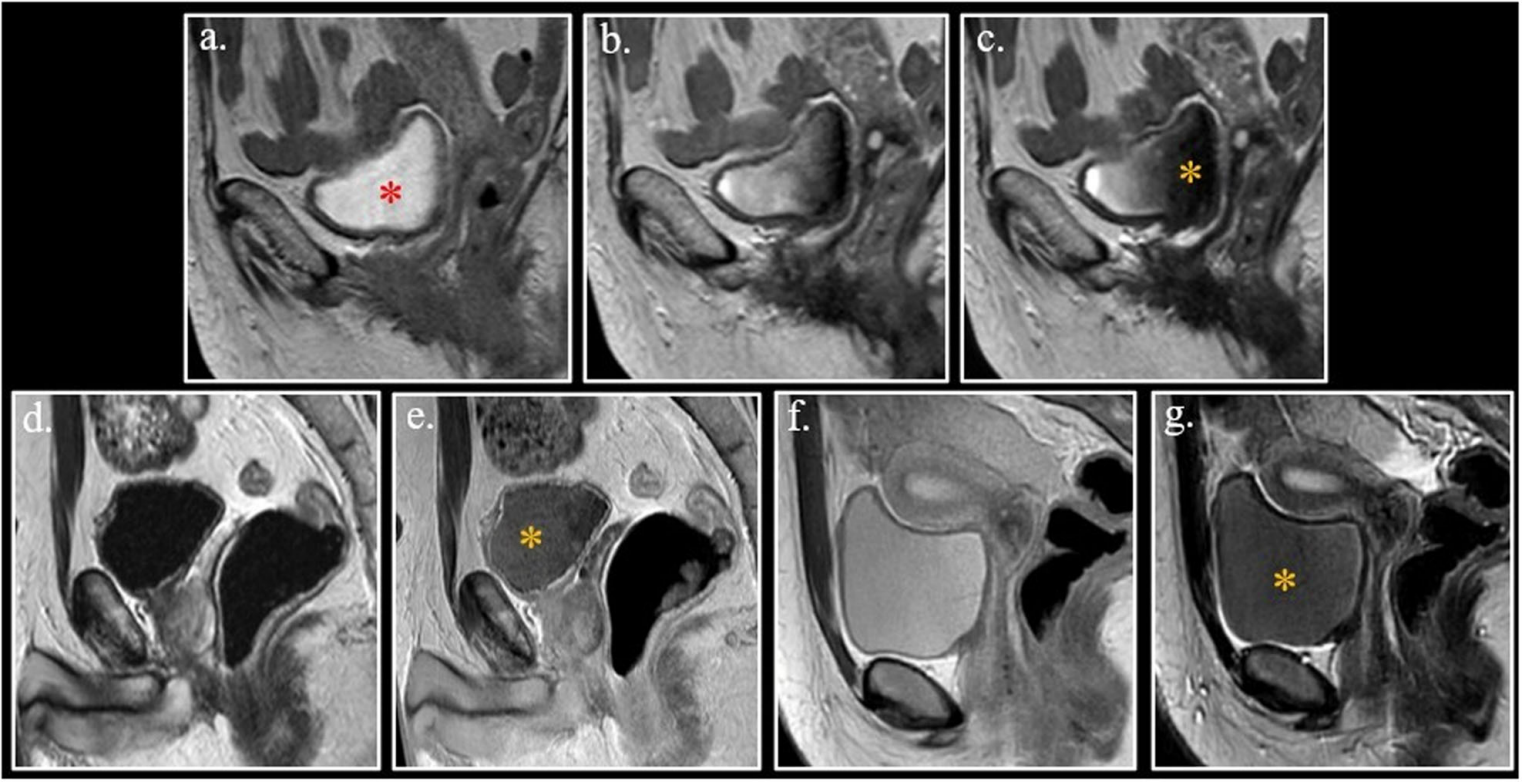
a–c On FSE T1-weighted sagittal image (a) of the bladder, homogeneous high signal of urine can be seen (red asterisk). A FSE T2-weighted image obtained immediately after T1 sequence shows a layering of Gadolinium. A lower strip of urine with more concentrated Gadolinium defines the so-called shading sign (b). After 15 minutes (c), the further concentration of Gadolinium causes enlargement of the shading sign (orange asterisk). d, e “Disappearance” of the urine signal on T2-weighted image due to diffuse high concentration of Gadolinium in the bladder. Sagittal T2-weighted (d) and T1-weighted images (e) after Gadolinium administration. The high concentration of Gadolinium causes the shading phenomenon on T2-weigthed image (d). In addition, there is a loss of signal of the urine also on T1-weighted image (yellow asterisk in (e)) because in this case the T2* of urine is longer than TE of T1 sequence, preventing the collection of MR signal. f, g A sagittal FSE double echo images at 40 ms (f) and 100 ms (g) through the bladder demonstrated the influence of different echo-times on the appearance of the shading sign. The shading sign (asterisk) can be seen only at TE 100 ms (g)
